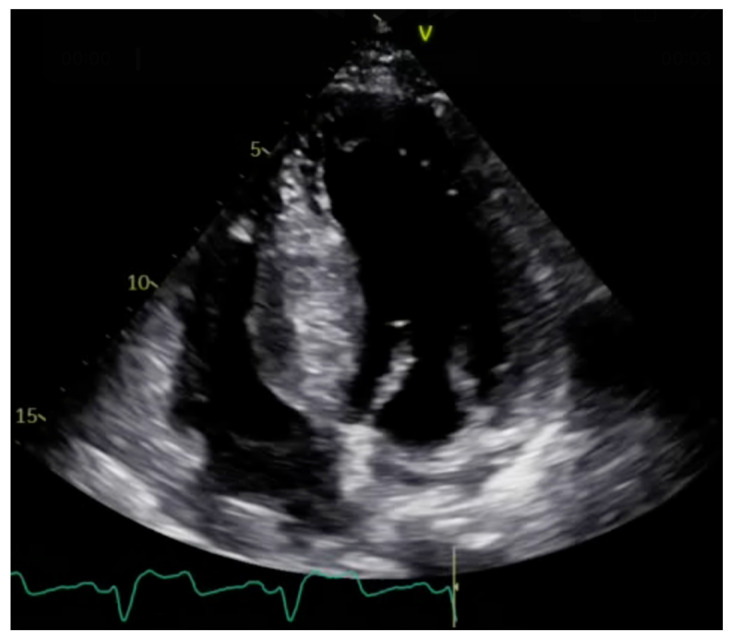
Transthoracic echocardiogram in patient with hypertrophic cardiomyopathy. Asymmetric septal hypertrophy.
